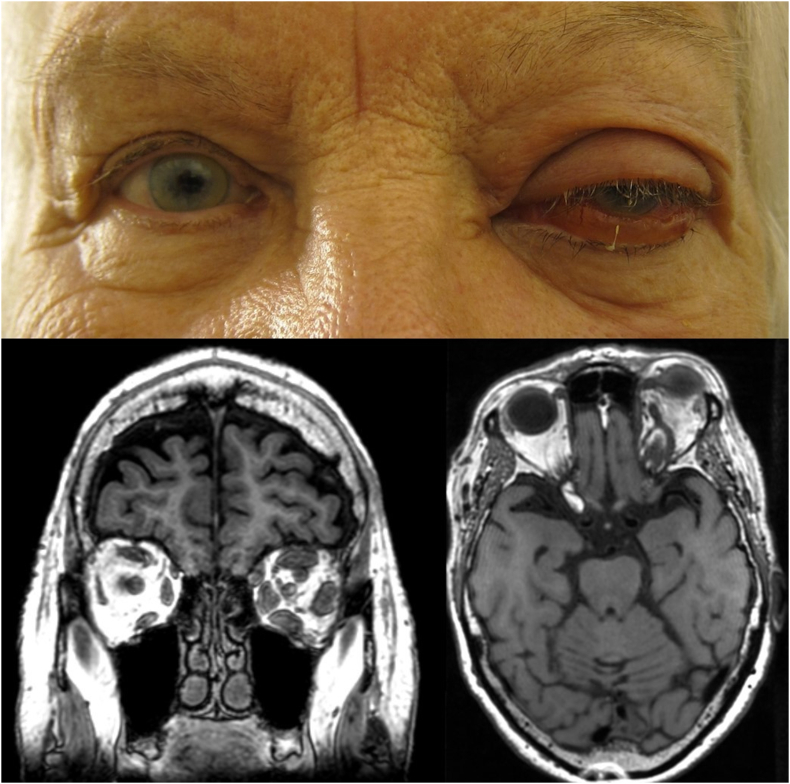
Case 2 – Patient with isolated superior ophthalmic vein thrombosis. A Photo at initial presentation. B–C Magnetic resonance imaging at initial presentation showing enlarged superior ophthalmic vein, orbital congestion and enlarged ocular muscles.
